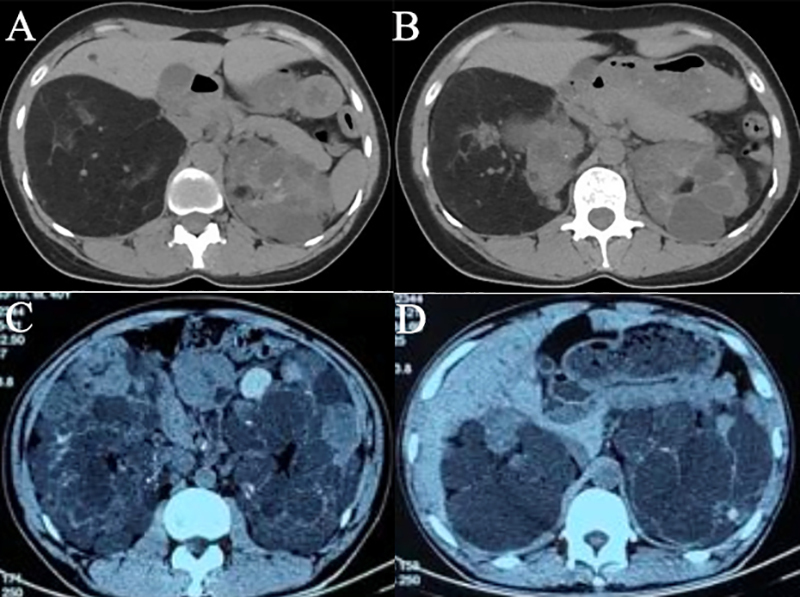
Computed tomography (CT) exam results: A and B, in a 35-year-old
